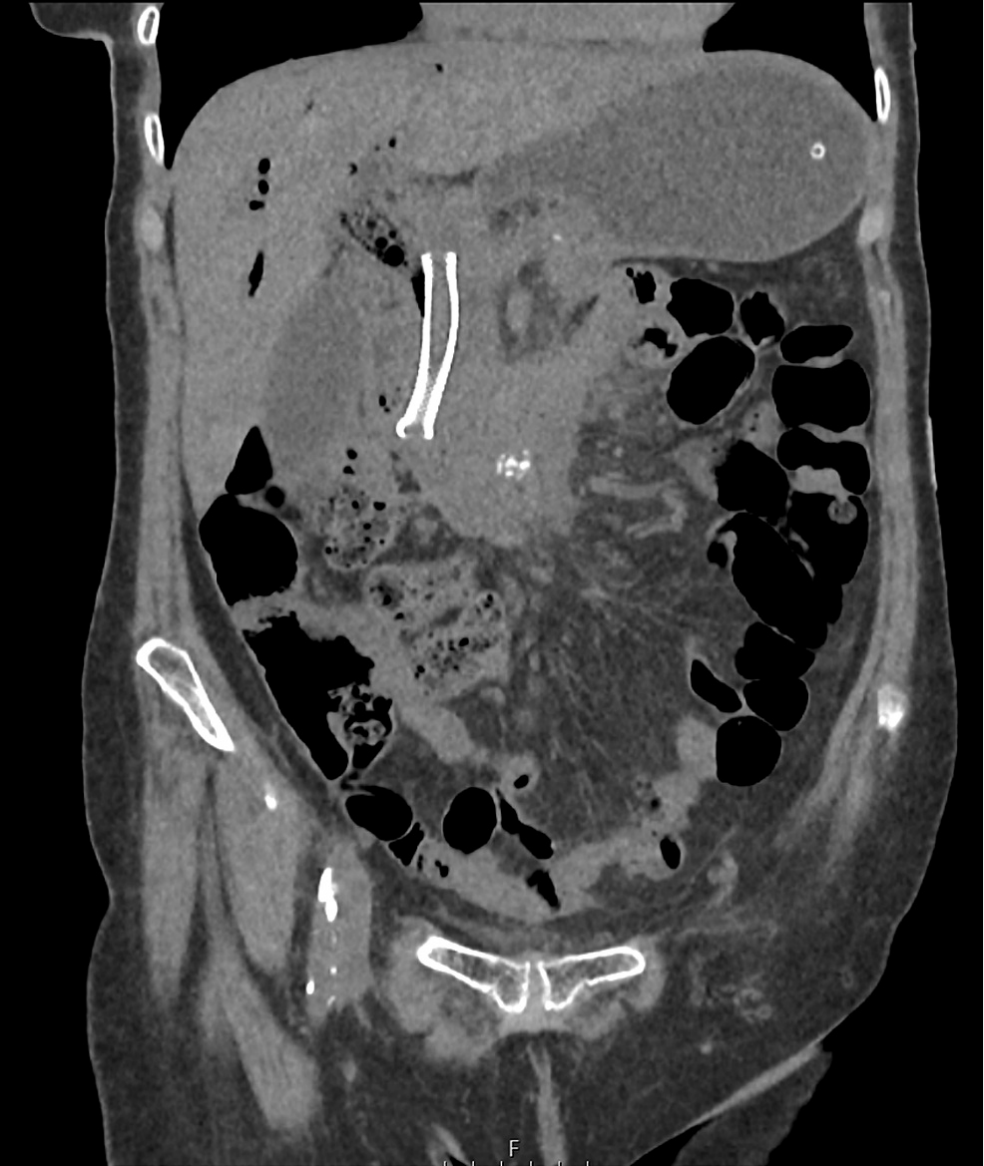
Computed tomography of the patient on admission: coronal plane
